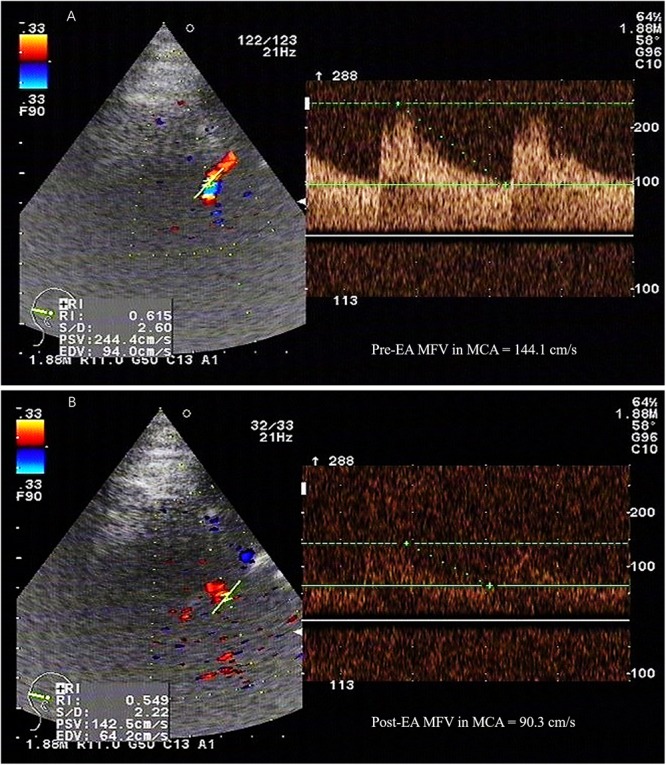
The MFV in the MCA was measured by TCD. PSV, peak systolic velocity; EDV, end-diastolic velocity; MFV, mean flow velocity; MCA, middle cerebral artery; TCD, transcranial doppler; EA, electroacupuncture. A 52-year-old female patient with subarachnoid hemorrhage caused by rupture of right anterior communicating artery aneurysm, developed vasospasm on the 5th day after surgery. The maximum value of flow velocity in systole was calculated at the apex of the waveform, which defined as PSV. EDV was measured at end diastole, and indicated the lowest point of waveform. MFV was calculated as EDV plus a third of the difference between PSV and EDV (MFV [cm/s] = [PSV + 2EDV]/3). TCD detection showed that the MFV in MCA in the range of 120∼140 cm/s was indicative of mild cerebral vasospasm, 140∼200cm/s was accord with moderate cerebral vasospasm, and above 200 cm/s was consistent with severe cerebral vasospasm. (A) TCD showed a high MFV in MCA (144.1cm/s) before EA. (B) The MFV in MCA (90.3cm/s) was decreased after EA.
